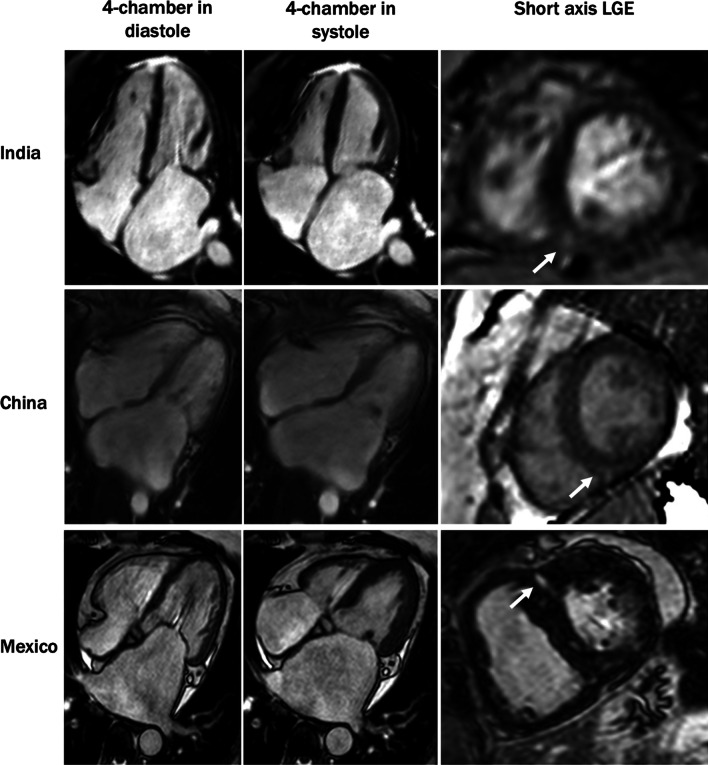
Representative examples of cardiovascular magnetic resonance images of patients with rheumatic mitral stenosis from India, China, and Mexico. White arrows point to regions of late gadolinium enhancement (LGE) at the right ventricular (RV) insertion sites
